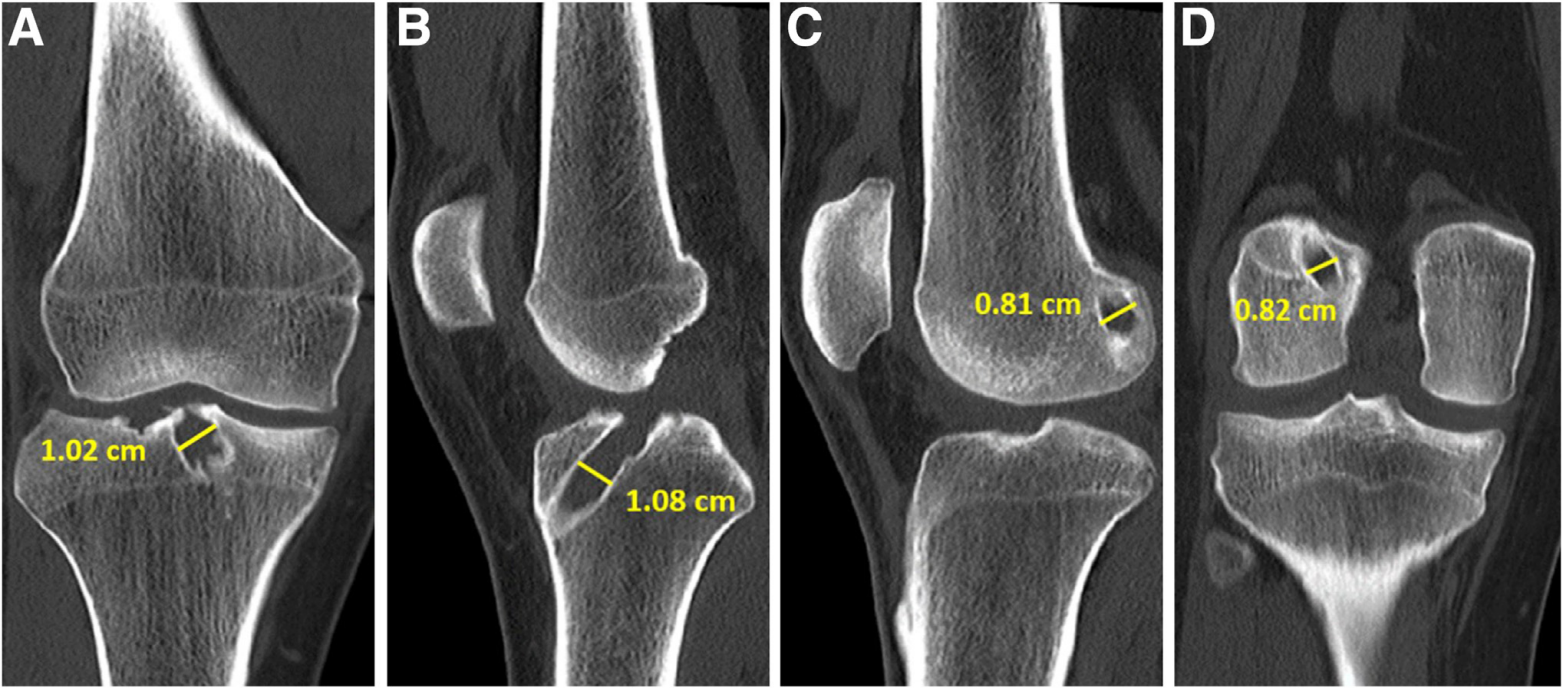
Computed tomography scans of the right knee in a patient with new terrible triad and ACL graft rupture. The scans show the measurement of the dimensions and position of the previous femur and tibia tunnels. (A) Coronal cut of tibia tunnel. (B) Sagittal cut of tibia tunnel. (C) Sagittal cut of femur tunnel. (D) Coronal cut of femur tunnel. (ACL, anterior cruciate ligament)
